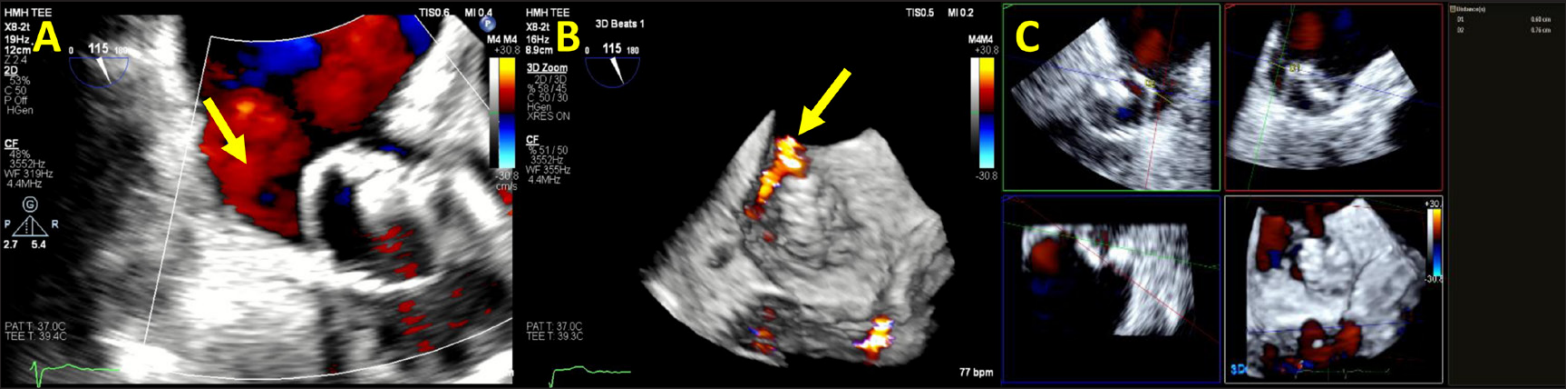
Transesophageal echocardiogram showing (A) Video clip of 2D color Doppler peri-Watchman device leak (yellow arrow). (B) Video clip of 3D color Doppler transesophageal echocardiogram showing peri-Watchman device leak (yellow arrow). (C) 2D multi-planer reconstruction of the Watchman device demonstrating the size of the peri-device leak at 0.8 × 0.6 cm. See Figure 2 A videoclip at https://youtu.be/LlqtscEZVR4 and Figure 2 B videoclip at https://youtu.be/F0qbwF45LLQ.
